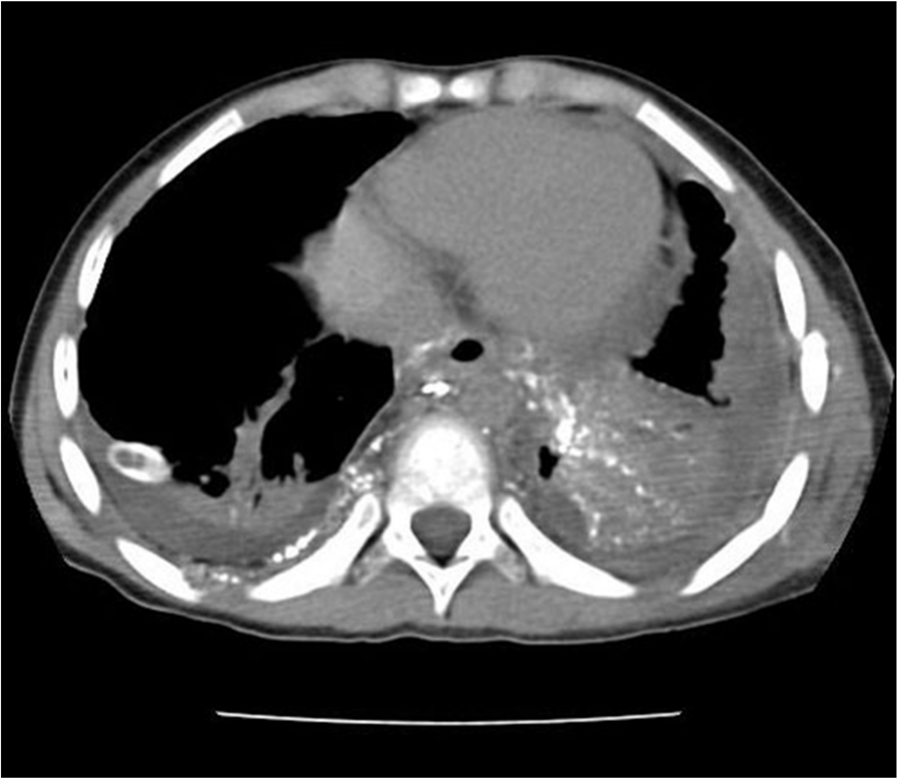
Extensive thoracic lymphatic leakage detected by post LAG non-contrast CT.
